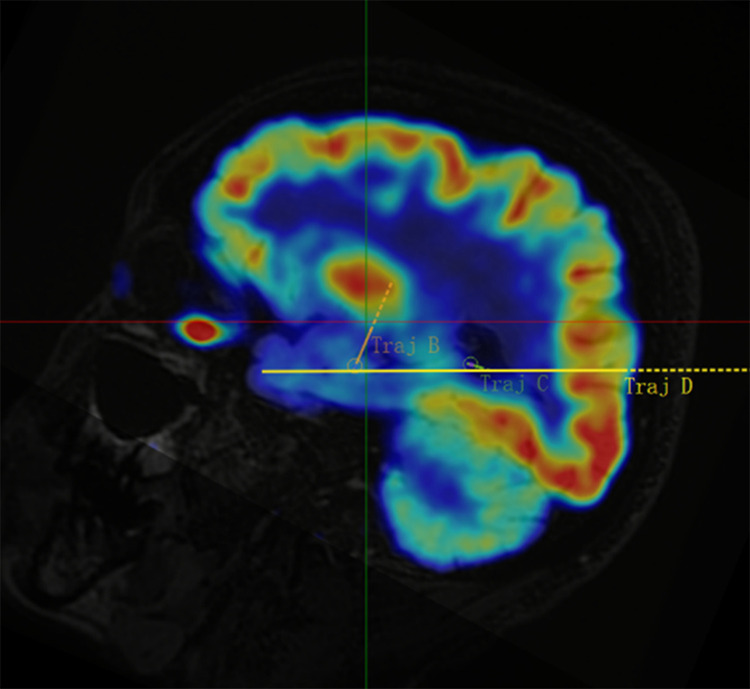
Co-registered sagittal MRI T2 LAIR and PET-CT images.
